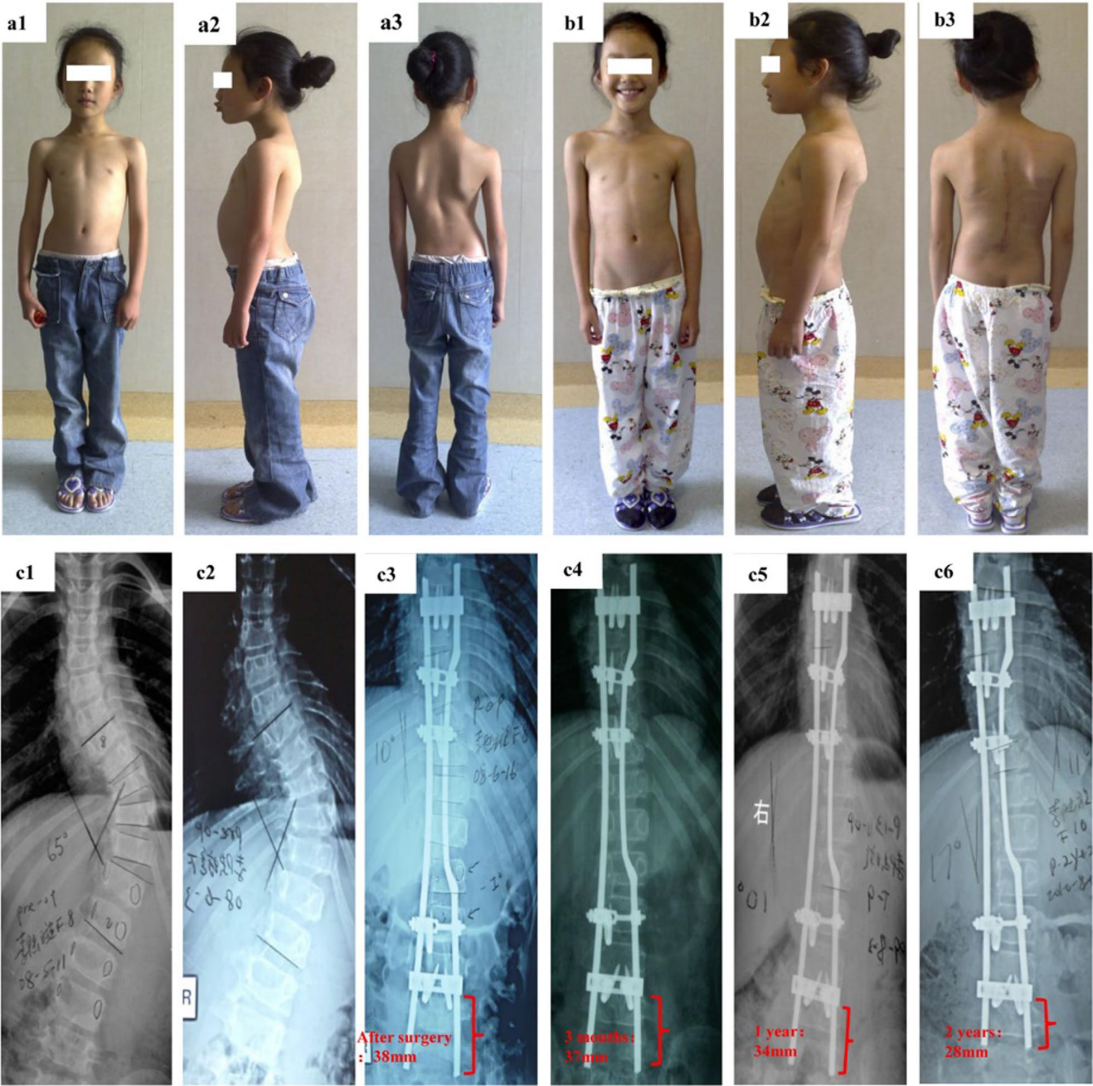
An 8-year-old girl with progressive early onset scoliosis before and after treatment with the plate-rod spinal system. a1–a3 Preoperative photographs. b1–b3 Postoperative photographs. c1 On plain radiographs, the preoperative Cobb angle is 65° before traction and c2 50° after traction. c3 The postoperative Cobb angle is 10° and the rod tail reserve length is 38 mm. c4 The rod tail length on plain X-ray at three months after surgery is 37 mm. c5 and c6 The rod tail length at one- and two years after surgery is 34 mm and 28 mm
